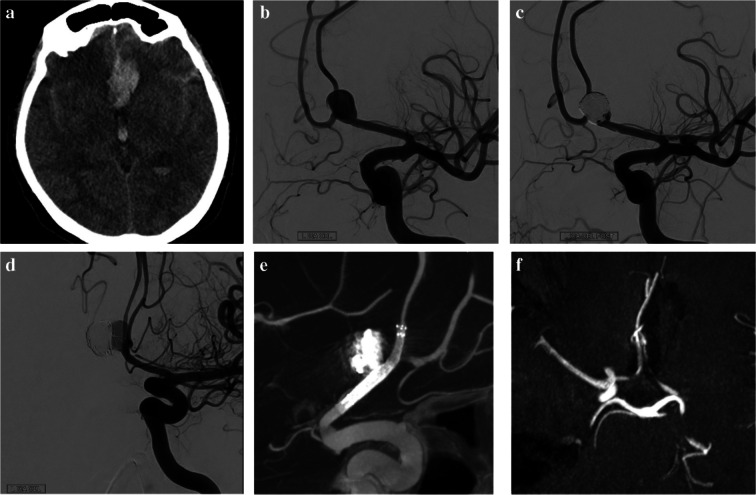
A 57-year-old female patient with SAH. (a) CT demonstrating cisternal and ventricular bleeding, along with a hematoma in the anterior interhemispheric sulcus. (b) Left carotid angiogram in a left oblique projection revealing a saccular aneurysm arising from the distal A1 segment of the left ACA. (c) After intrasaccular coil deposition with the assistance of an LVIS Evo stent deployed in the A1 and A2 segments of the left ACA, the final angiogram shows some residual filling. (d) Two and a half months later—15 days prior to the scheduled follow-up imaging—the patient experienced another SAH. A left carotid angiogram in a slight contralateral oblique projection reveals regrowth of the aneurysm. (e) Cone-beam CT imaging following placement of an additional LVIS Evo stent and subsequent SVB implantation. (f) MR examination six months later reveals no aneurysmal filling and patent parent vessels
